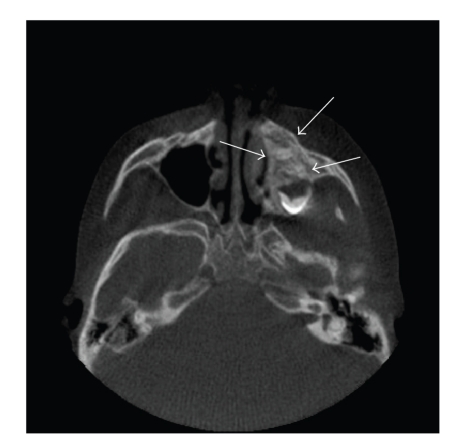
Axial cone beam computed tomography view showing left maxillary alveolar expansile lesion with a dystrophic, ill-defined trabecular pattern.
